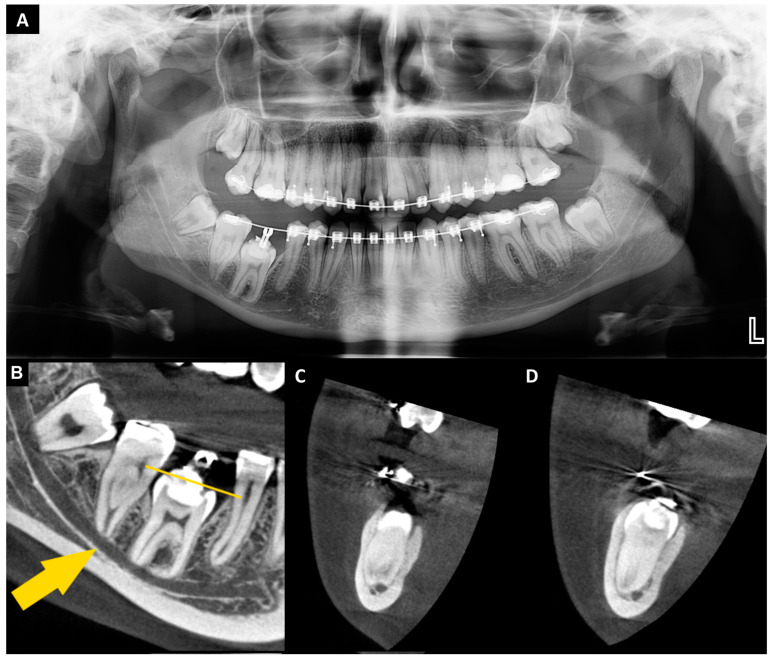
Orthopantomography (A) reincluded tooth no. 46 and donor—tooth no. 38; cone beam computed tomography (B–D) (radiological examinations performed 1 month before the procedure): (B) sagittal view—severe reinclusion of tooth no. 46 (yellow line—the crown of the tooth located under the mucous membrane); yellow arrow—inferior alveolar nerve canal); (C) cross-sectional view—mesial root of tooth no. 46 in relation to IANC; (D) cross-sectional view—distal root of tooth no. 46 in relation to IANC.
